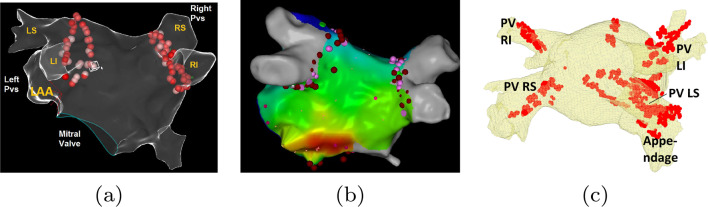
Clinical use of LA surface. a LA anatomy and typical ablation (red points), b LA electric propagation map. c Initial bearing path in red, CT anatomy is in yellow. Most of the red points lie inside the blood pool (not over the surface)
